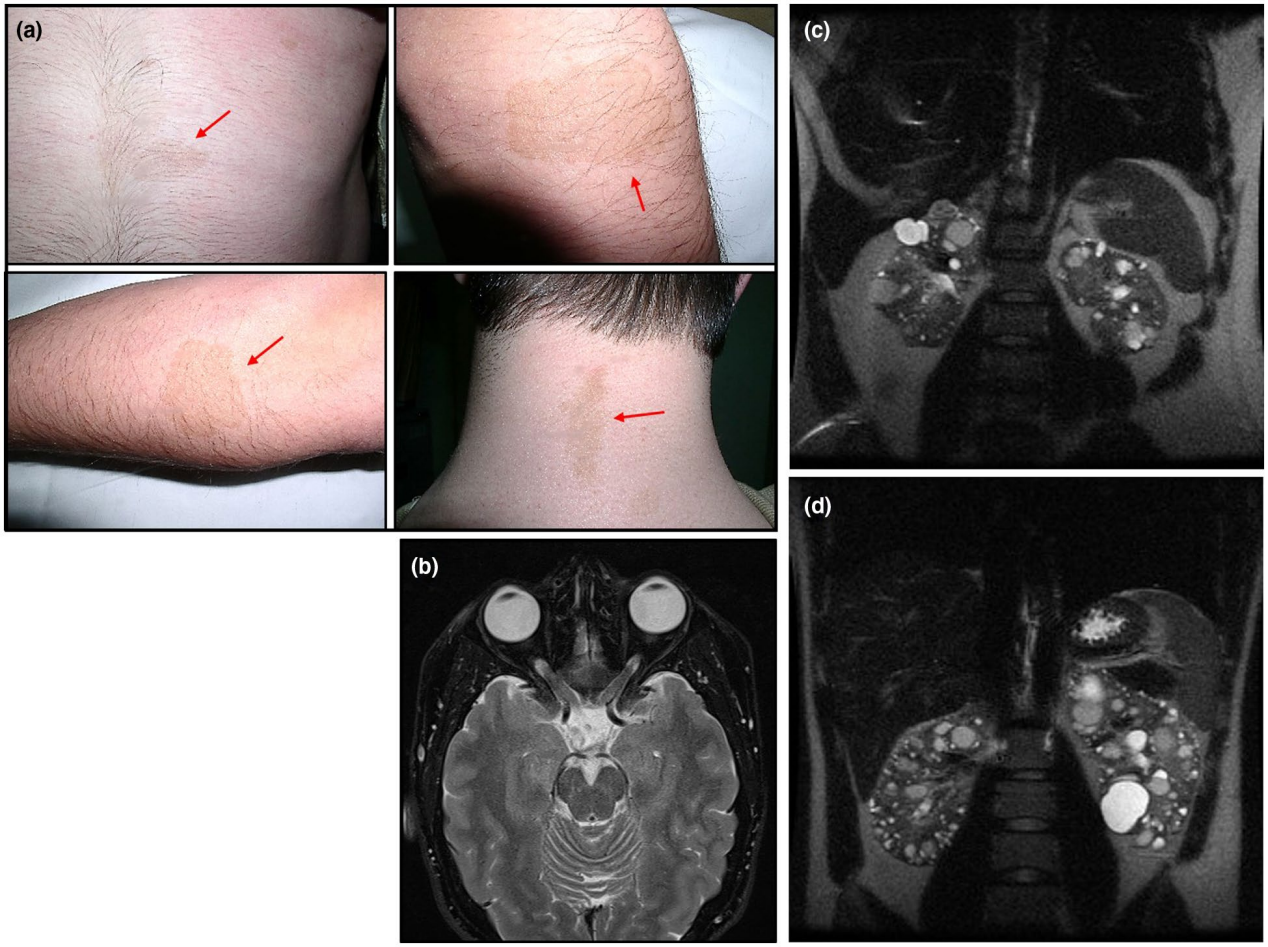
Clinical features for concurrence of NF1 and ADPKD in the proband. (a) Presence of café‐au‐lait spots over neck, trunk, and extremities. (b) Axial MRI at the age of 17 years showing bilateral thickening of the optic nerve and chiasm. There were focal areas of increased signal intensity on T2 in the bilateral globus pallidus and hippocampus. (c) Coronal T2‐weighted MRI scan of the abdomen in the proband at the age of 28 years showing a TKV of 587 ml (htTKV of 360 ml/m). (d) Coronal T2‐weighted MRI scan of the abdomen in his brother at the age of 30 years showing a TKV of 791 ml (htTKV of 437 ml/m). ADPKD, Autosomal dominant polycystic kidney disease; htTKV, height adjusted total kidney volume; MRI, magnetic resonance imaging; NF1, neurofibromatosis type 1; TKV, total kidney volume
